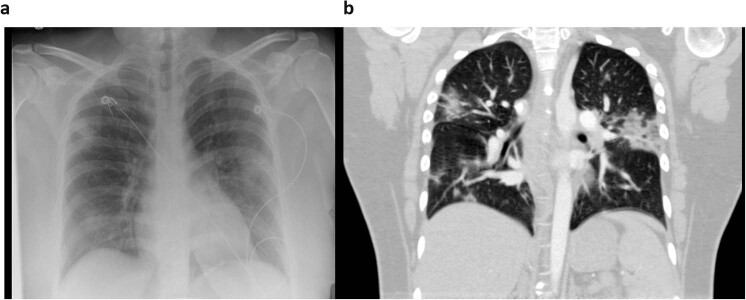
Chest Radiograph (a) and Computed Tomography (CT) image in the sagittal plane (b) of the same patient (Covid-19-Ar-16434363) taken on the same day. Patchy airspace opacities and ground-glass changes are seen in both lungs.
